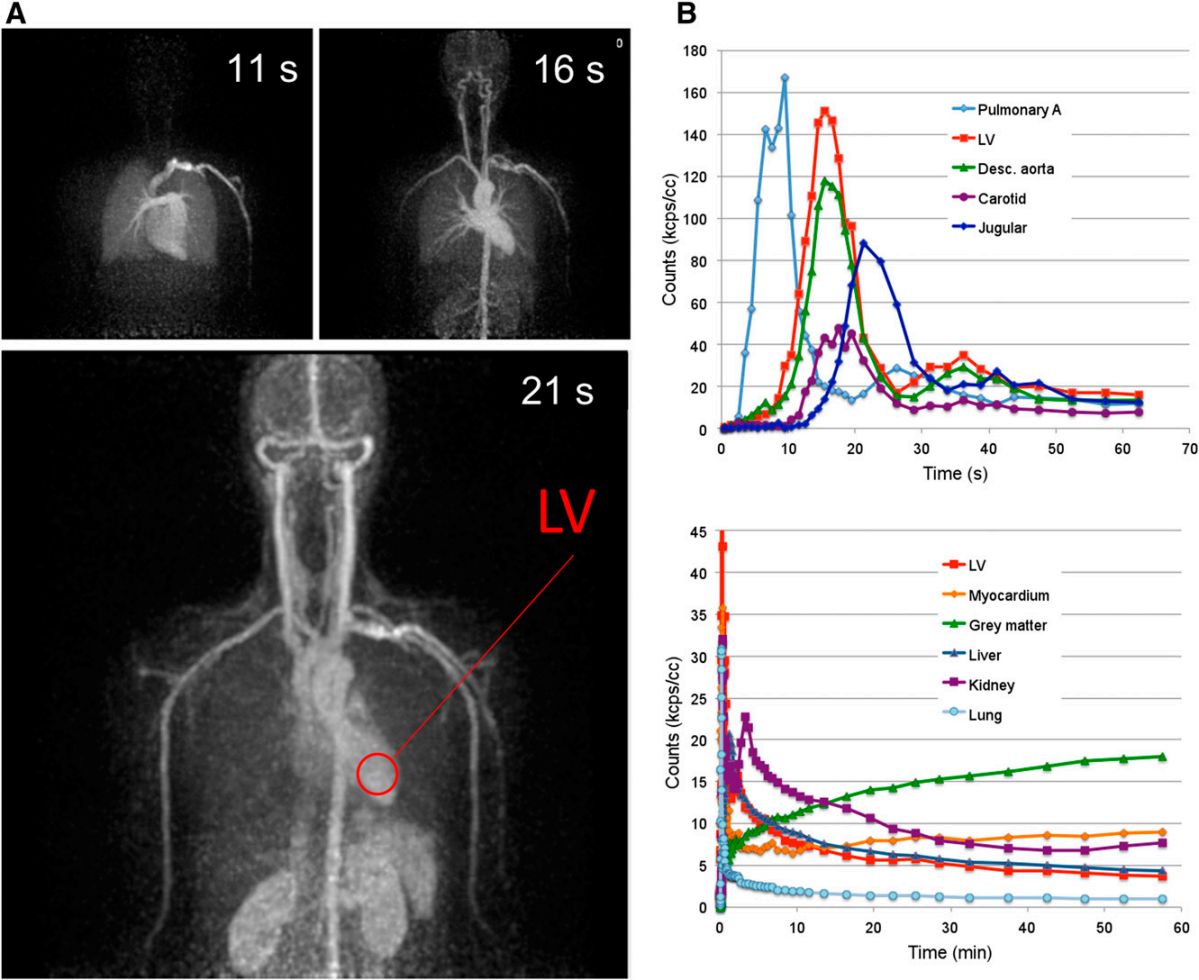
(A) ^18^F-FDG PET maximum-intensity projections of subject 7, each 1 s in duration, at 3 time points from dynamic scan. (B) Time–activity curves of blood input function measured at several vessels over first minute after injection, and time–activity curves of major organs over first hour after injection. LV = left ventricle.
